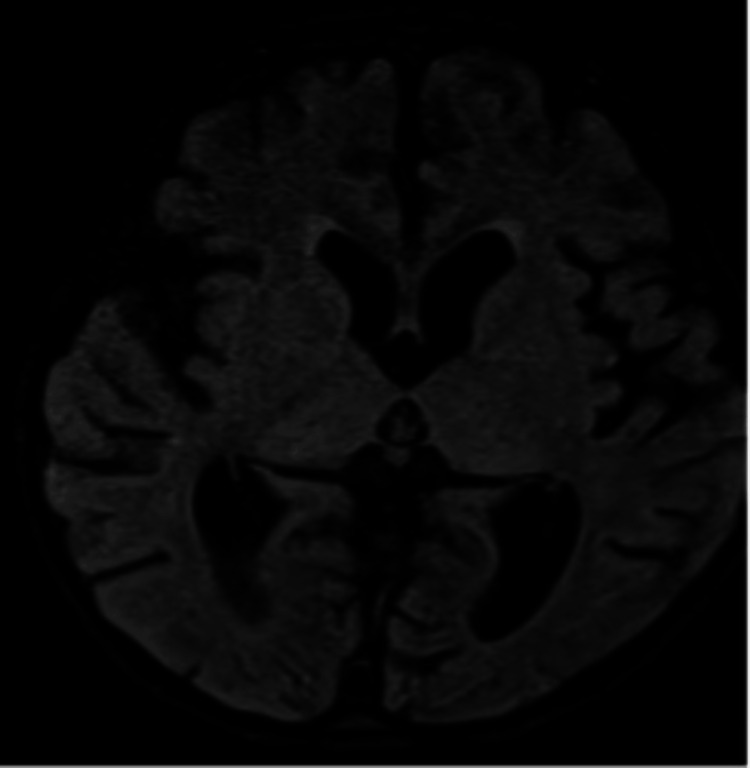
MRI showing involvement of thalami and diffuse cerebral atrophy in a case of rabies encephalitis included in our study All three MRI pictures were of participants included in our study. MRI: magnetic resonance imaging
